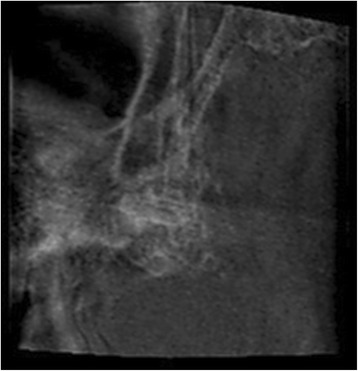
Motion artefact evident in CBCT image of the posterior region of the right jaw with 5 × 5 FOV and 12.01-s acquisition time
